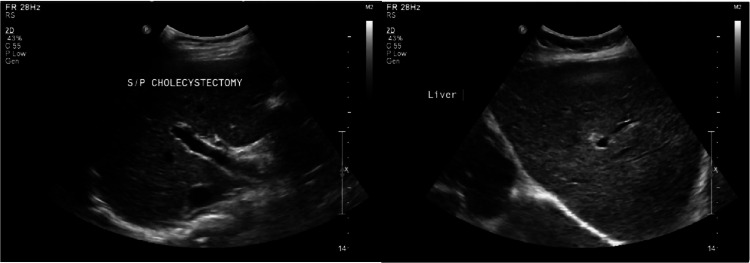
Ultrasound of the liver showing homogeneous echotexture and unremarkable postcholecystectomy biliary anatomy
